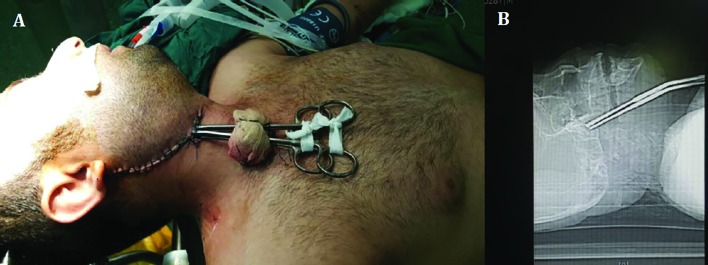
The intraoperative image of the patient demonstrating temporary closing of the wound with two vascular clamps in place for bleeding control (A); Scot image of the computed tomography (CT) scan of the patient demonstrating the clamps in place
